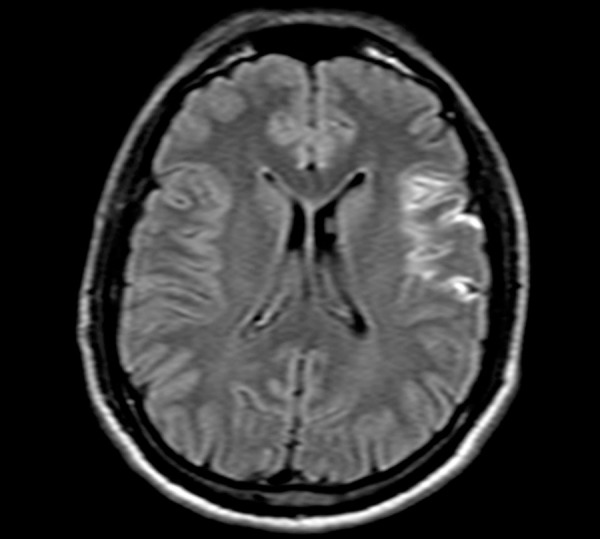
Brain Magnetic Resonance Imaging (FLAIR) in axial section showing
